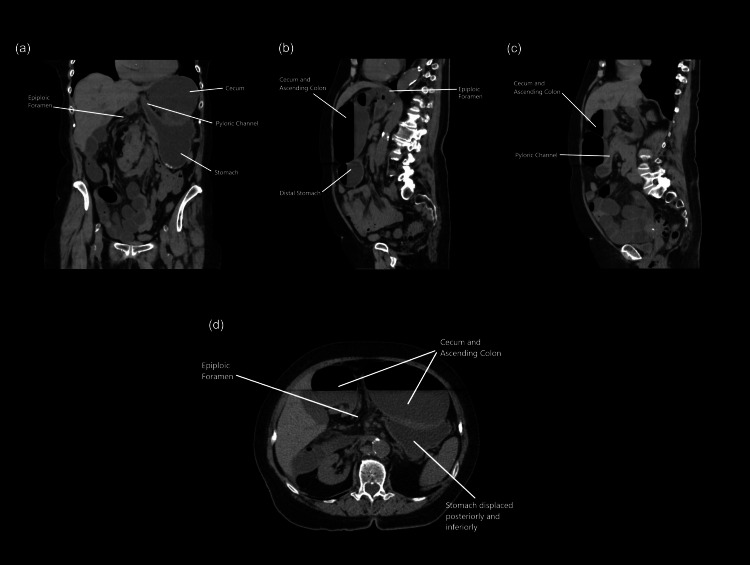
CT abdomen and pelvis without contrast (a-d). The cecum is shown herniating through the epiploic foramen of Winslow and posterior to the pyloric channel.
